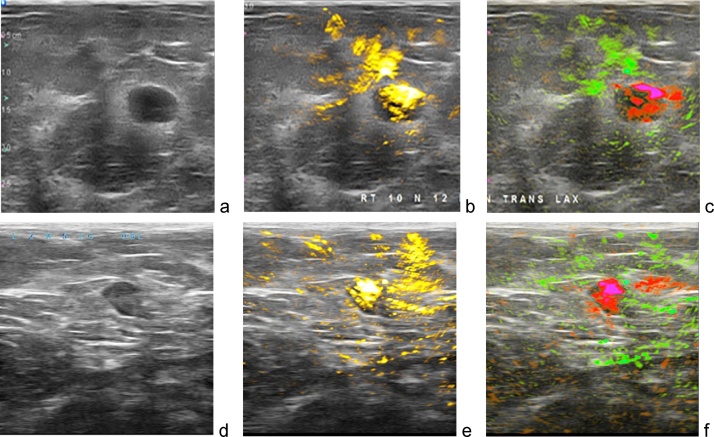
Two examples of OA/US images changing the diagnosis from probably benign solid masses to masses suspicious for carcinoma. Optoacoustic functional images upgraded radiologist interpretation to BI-RADS 5 (highly suspicious for malignancy) from BI-RADS 3 (probably benign) based on conventional ultrasonic morphological images. Biopsy confirmed malignancy in both patients. Images are 38 × 38 mm.
